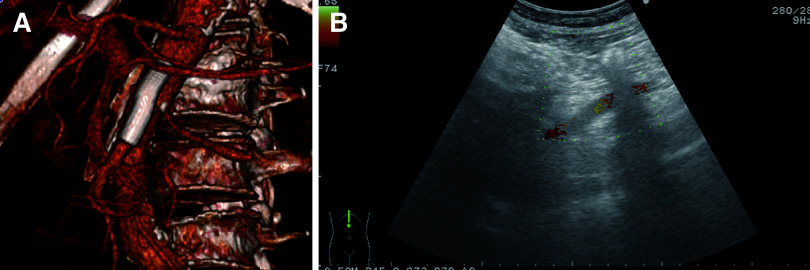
Fig. 3 (A) Postoperative CT showed exclusion of the aneurysm, patent stent-graft, and preservation of the middle colic artery as well as the distal branches of the SMA with no endoleak. (B) Postoperative echo at 3-year follow-up showed patent stent-graft of SMA. CT: computed tomography; SMA: superior mesenteric artery.
